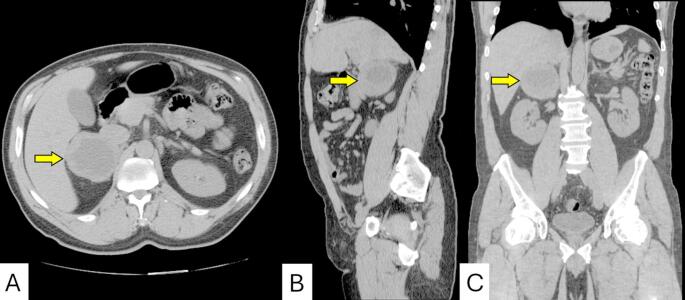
Non-contrast CT of the abdomen in axial (A), sagittal (B), and coronal (C) views demonstrating a large right adrenal mass (yellow arrows). The lesion is well-defined and heterogeneous, measuring approximately 7 cm, with areas of low attenuation suggestive of internal necrosis
